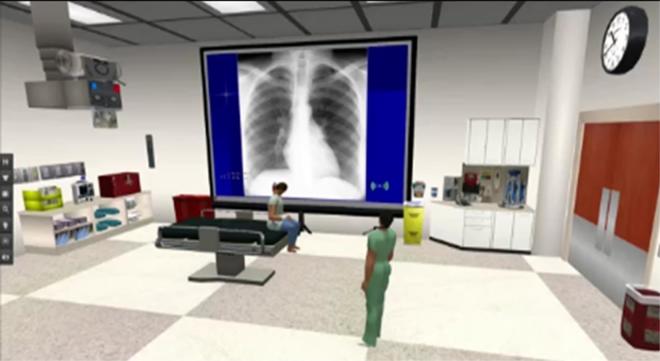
Physician (examinee) avatar examining a patient in an emergency department examination bay after requesting chest radiograph.
